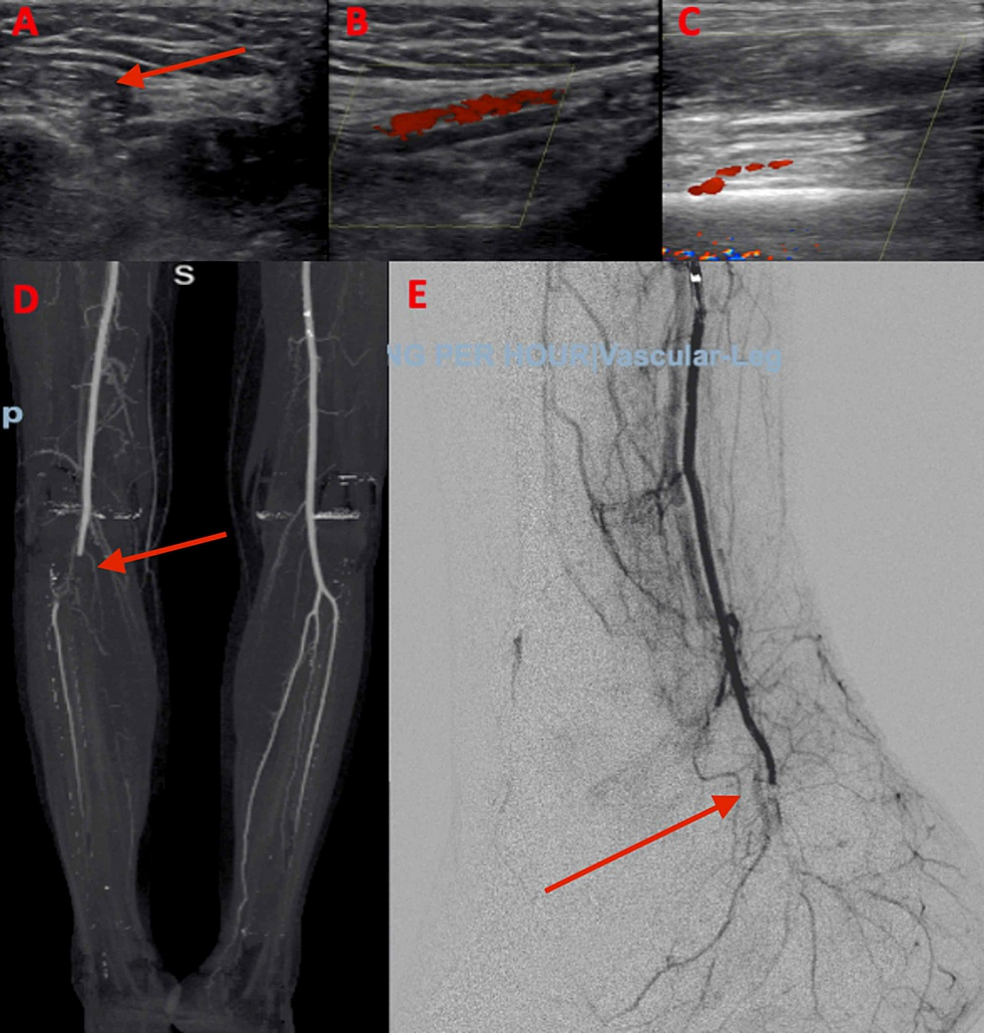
Arterial occlusion in COVID-19 infection. A-B: Arterial duplex of the right posterior tibialis showing the clot with no distal flow. C: Arterial duplex of the right dorsalis pedis with occlusion. D: CT angiography showing occlusion at the junction of the popliteal and peroneal posterior tibial trunk. E: Angiogram showing no flow to the distal right foot.
